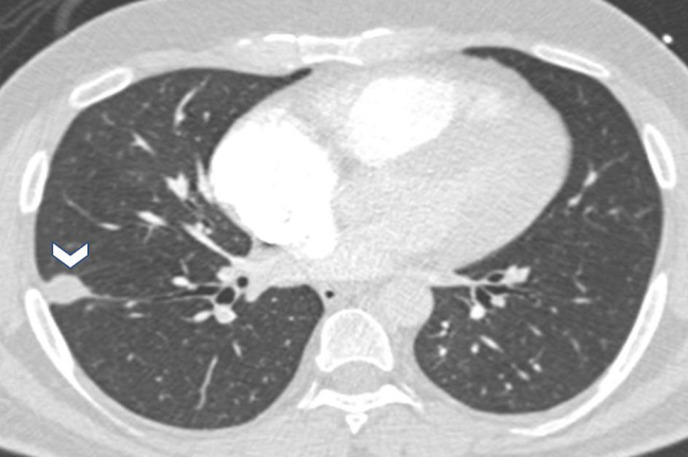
CT pulmonary angiogram axial lung window demonstrating a well-defined right lung lateral opacity (White arrow head)
